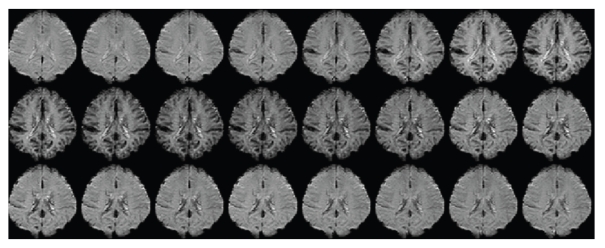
Perfusion MR images from a 39-year-old volunteer. Images encompass the first circulation (7–30, from left to right, top to bottom) at an upper slice location.
